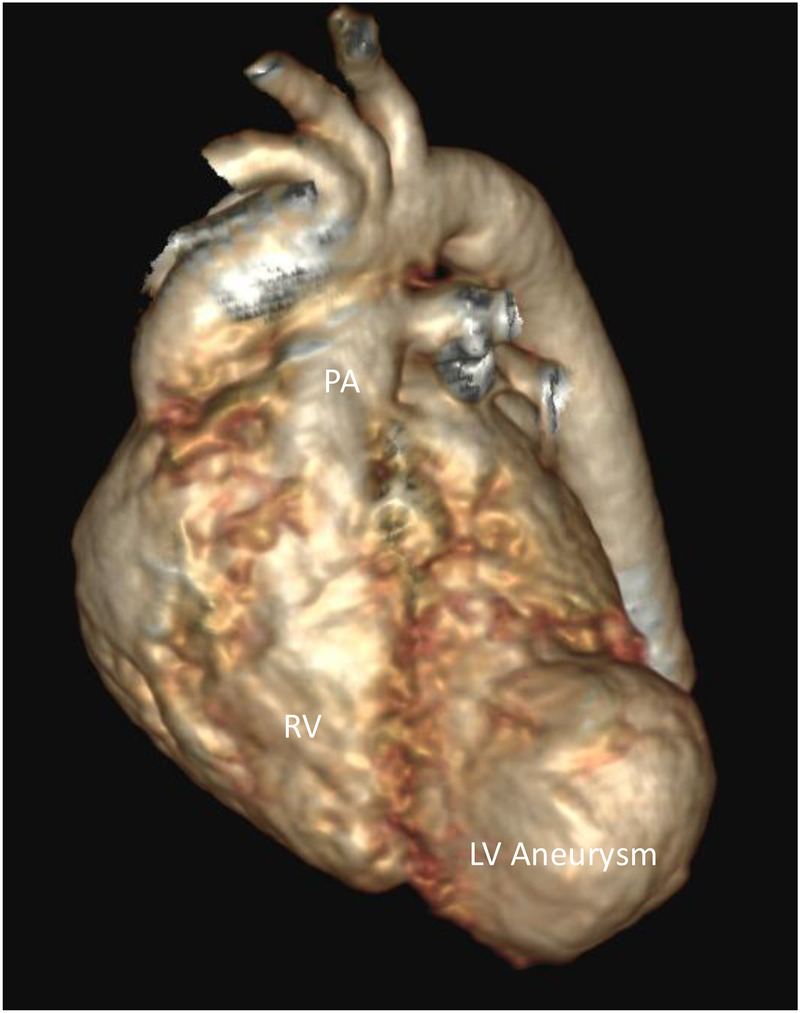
Constructed 3D cardiac CT showing a left ventricular apical aneurysm. RV, right ventricle; PA, pulmonary artery; LV, left ventricle.
